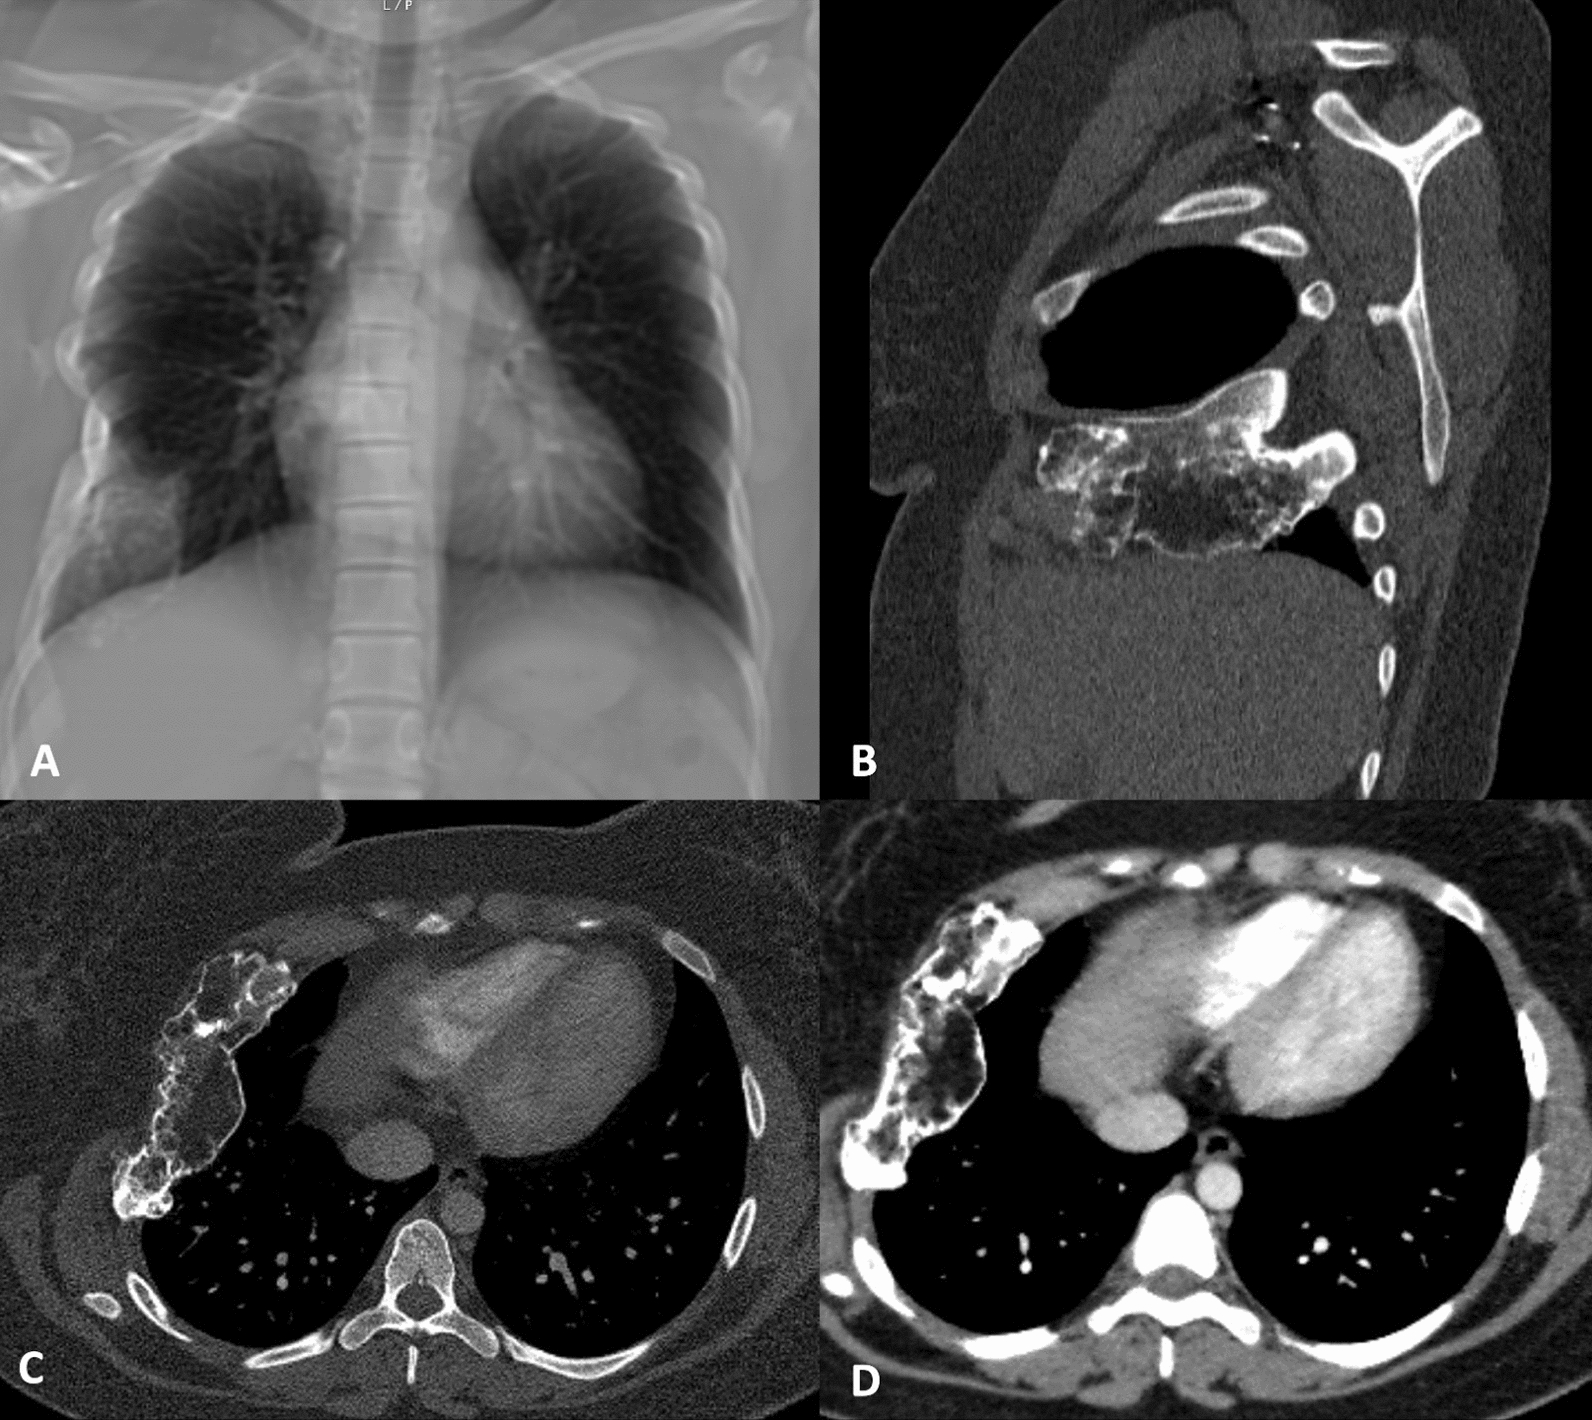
Chest X-ray (A) showing significant deformity of the rib cage on the right. Sagittal (B) and axial (C and D) sections of chest computed tomography demonstrating a lesion involving the fifth and sixth ribs, with an inflatable, lytic appearance with dense areas, including an aspect of ground glass, without an evident component of cortical rupture or associated soft tissue mass.; lesion measured 10.4 × 3.7 cm
